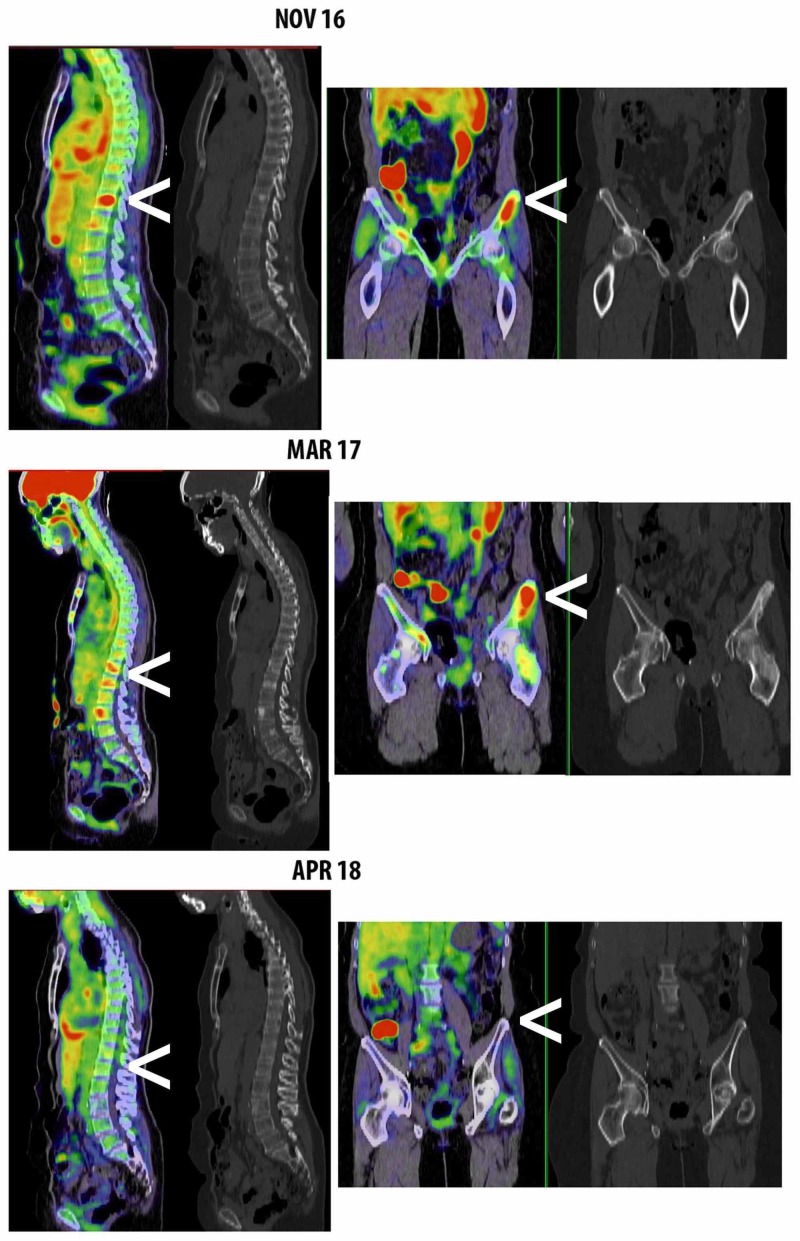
Serial PET-CT imaging Sagittal and coronal views of positron emission tomography-computed tomography (PET-CT) show initial progression of disease (from November 2016 to March 2017) and subsequent response (from March 2017 to April 2018) to immune therapy after infection and treatment of cytomegalovirus (CMV) with select areas indicated by white arrows.
